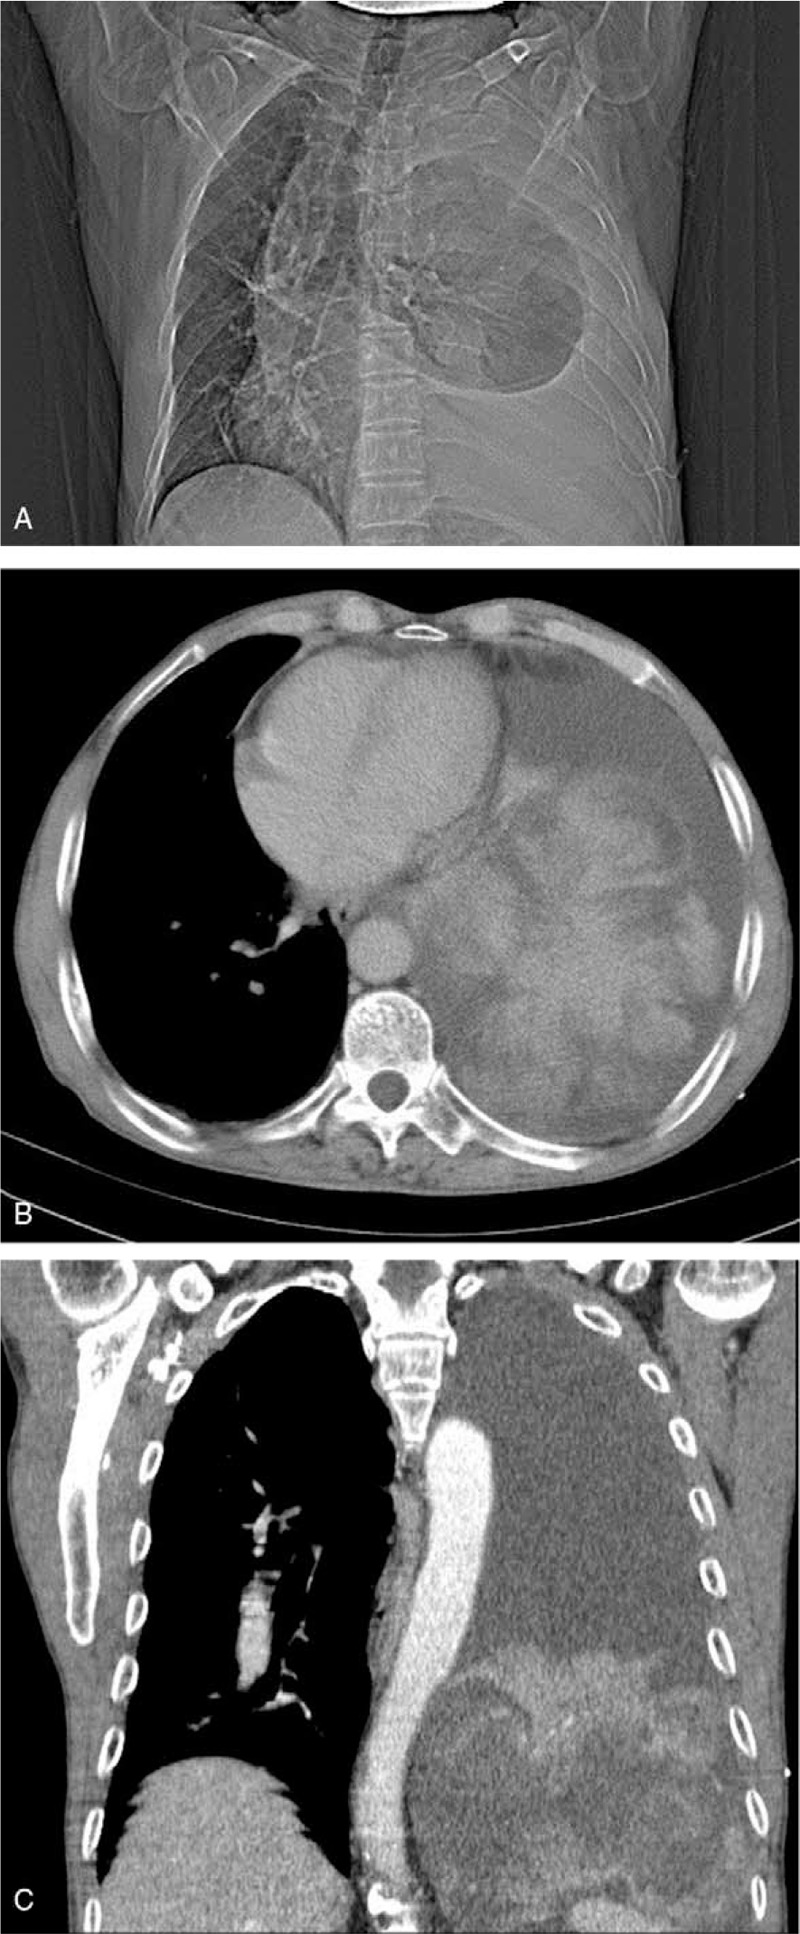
A1. X-ray showed that the pleural effusion seriously compressed the left lung and the mediastinum organs, the aorta and the trachea were extremely shifted to the right side. A2-A3. CT scan showed clear border, irregular lobular shadow and hemorrhagic-necrotic foci after enhancement, along with twisted vascular shadow.
